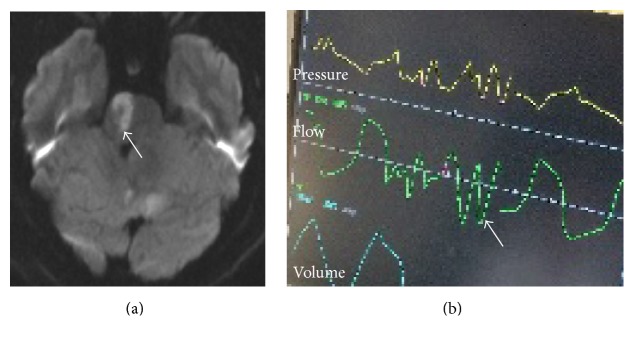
Magnetic resonance imaging of the brain. (a) Diffusion weighted imaging (DWI) showing right acute pontine ischemia (arrow). (b) Ventilator picture showing ataxic breathing pattern (arrow).
